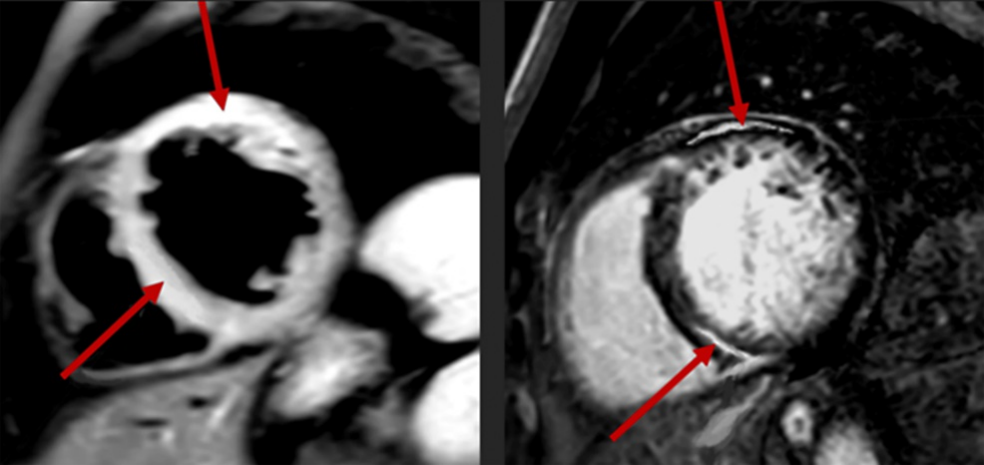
MRI of the heart showing hyperintense signal on the T2-weighted image (left) and delayed gadolinium enhancement on the T1-weighted image (right) indicating active inflammation in the anterior and inferoseptal walls of the left ventricle (arrows). The right ventricle myocardium was also involved but to a much lesser extent (not shown). These findings are consistent with acute autoimmune myocarditis.
